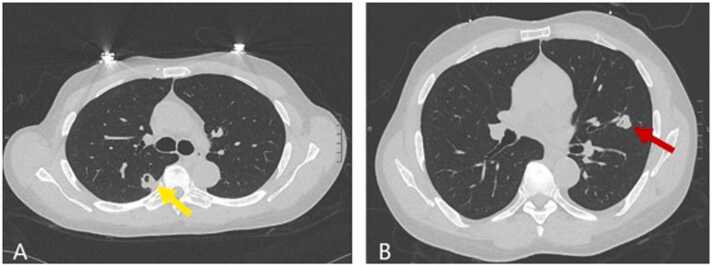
CT scan of the chest without IV contrast. A: Cavitary pulmonary mass in the superior segment of the right lower lobe measuring approximately 15 × 18 mm (yellow arrow). B: cavitary mass in the posterior left upper lobe measuring 15 × 12 mm (red arrow).
